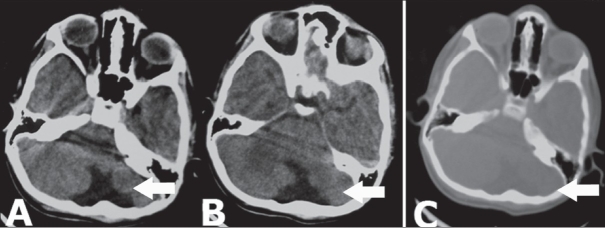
Axial CT scan images show hypoplasia of the left cerebellar hemisphere (arrows in A,B) with asymmetry of the posterior fossa (arrow in C)
